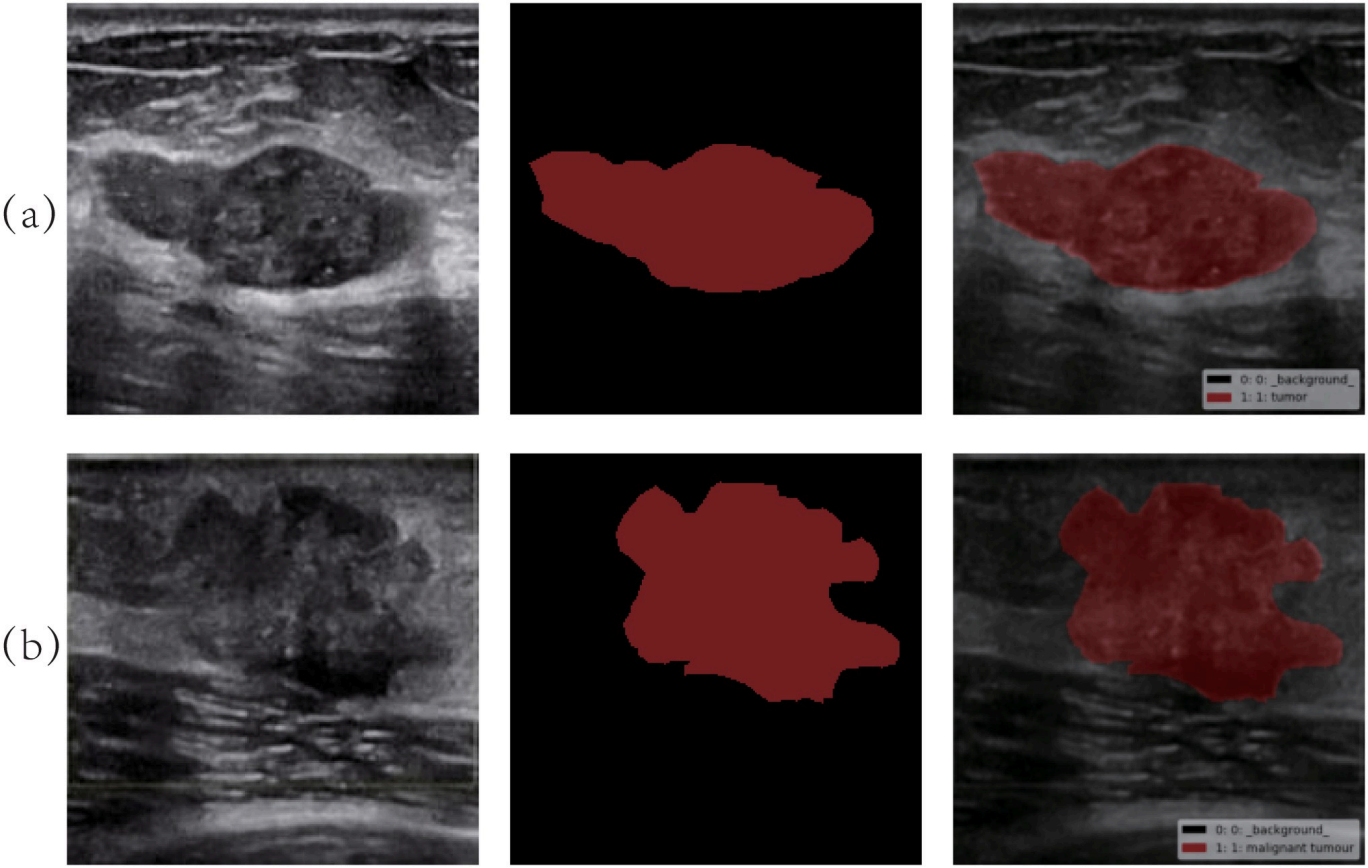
Ultrasound image and label of breast tumour. Picture (a) is a benign tumour, and picture (b) is a malignant tumour.
